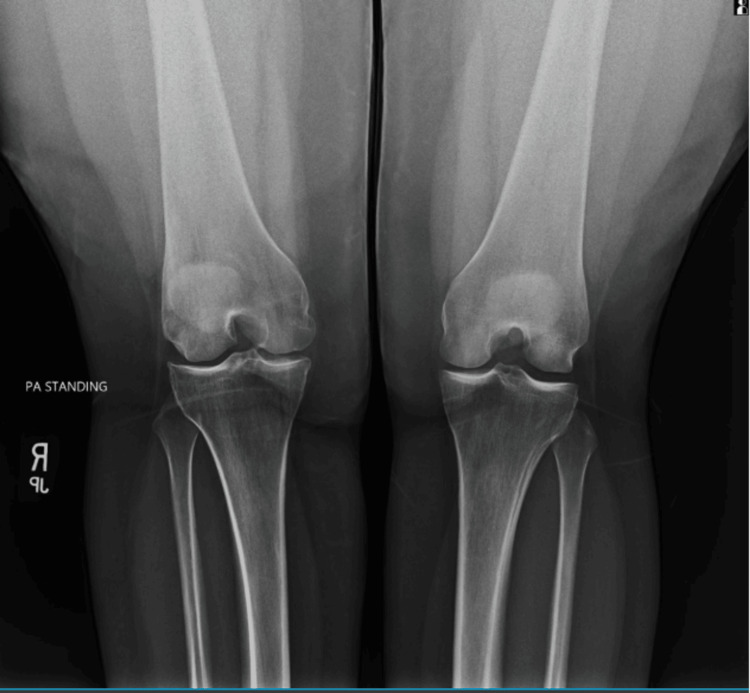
Weight-bearing posteroanterior radiograph of both knees demonstrating asymmetric lateral compartment joint space narrowing
